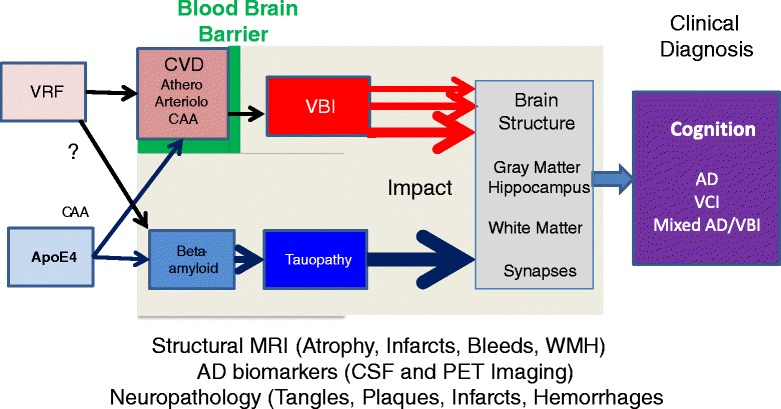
Mixed Alzheimer disease/vascular brain injury. Additive parallel or interactive pathways? AD, Alzheimer disease; ApoE, apolipoprotein E; CAA, cerebral amyloid angiopathy; CSF, cerebrospinal fluid; CVD, cerebrovascular disease; MRI, magnetic resonance imaging; PET, positron emission tomography; VBI, vascular brain injury; VCI, vascular cognitive impairment; VRF, vascular risk factor; WMH, white matter hyperintensity.
